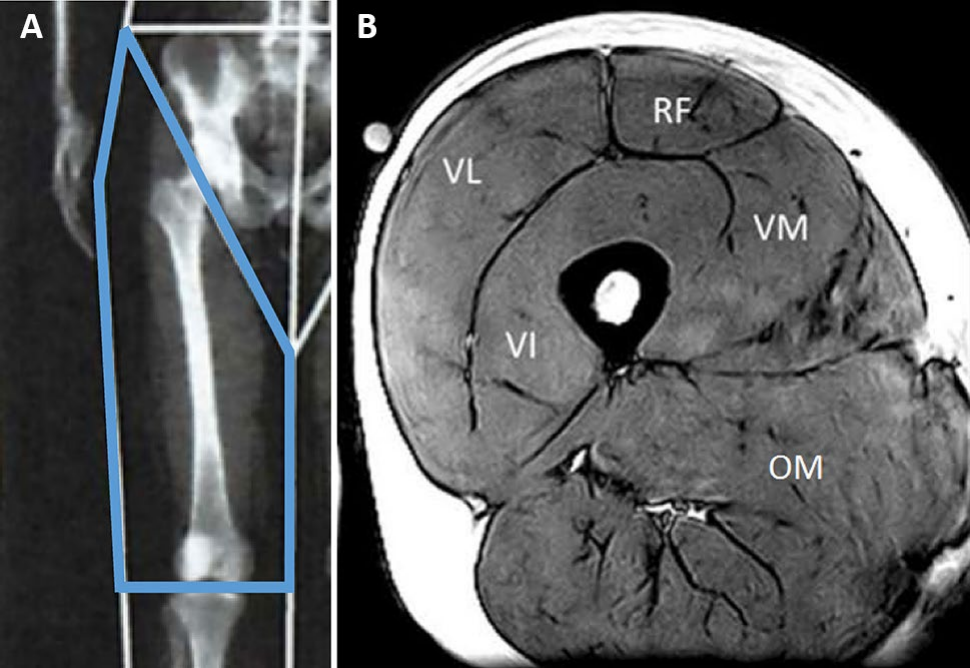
a Example of dual-energy X-ray absorptiometry (DXA) image showing regions of interest of the thigh. b Magnetic resonance imaging (MRI) image of the thigh muscles. *VI* vastus intermedius, *VL* vastus lateralis, *VM* vastus medialis, *RF* rectus femoris, *OM* other muscles
